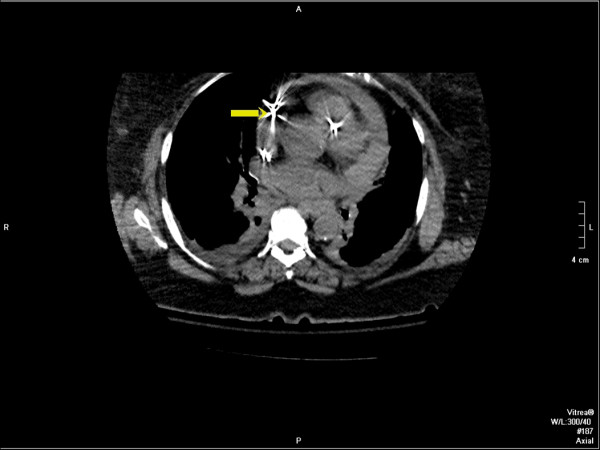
CT scan coronal reformatted image from an unenhanced CT of the chest demonstrates dense pericardial fluid consistent with hemopericardium. Note the pericardial fluid is isodense to the blood in the left ventricle.
